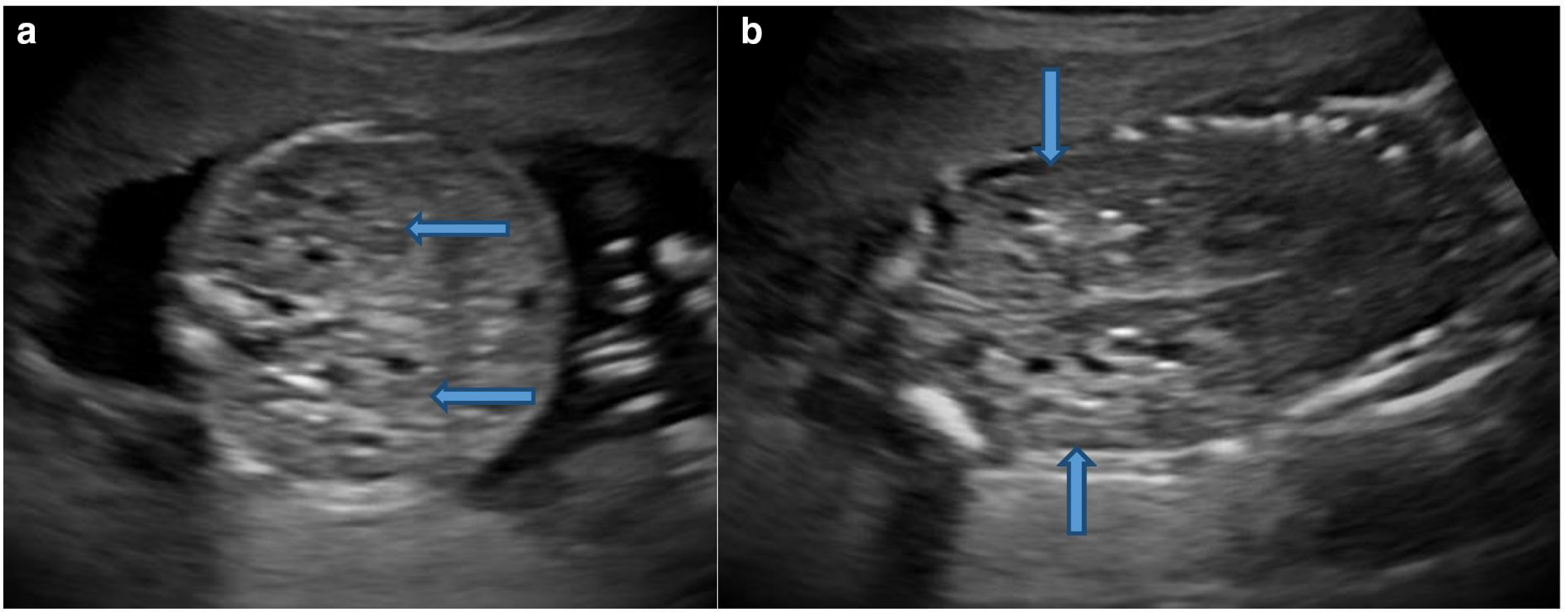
Ultrasound. Axial section (A) and Coronal section (B) of the abdomen showing bilateral enlarged and echogenic kidneys with few small cysts (block arrows).
